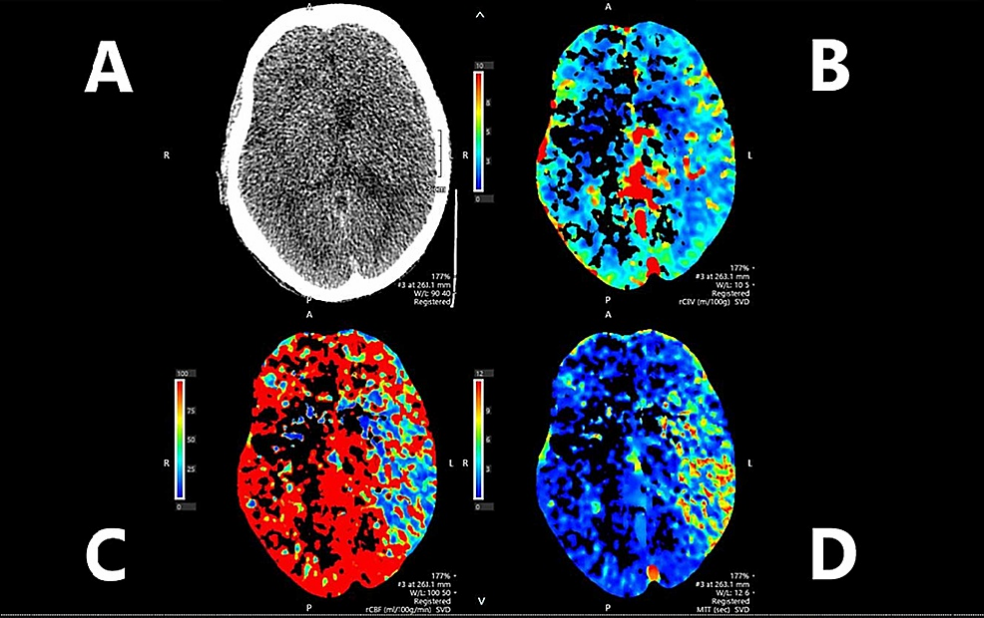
CT perfusion Non-contrast CT head (A), cerebral blood volume (B) showing relatively preserved blood volume, cerebral blood flow (C) showing reduced blood flow, and mean transit time (D) showing increased time within the left cerebral hemisphere.
